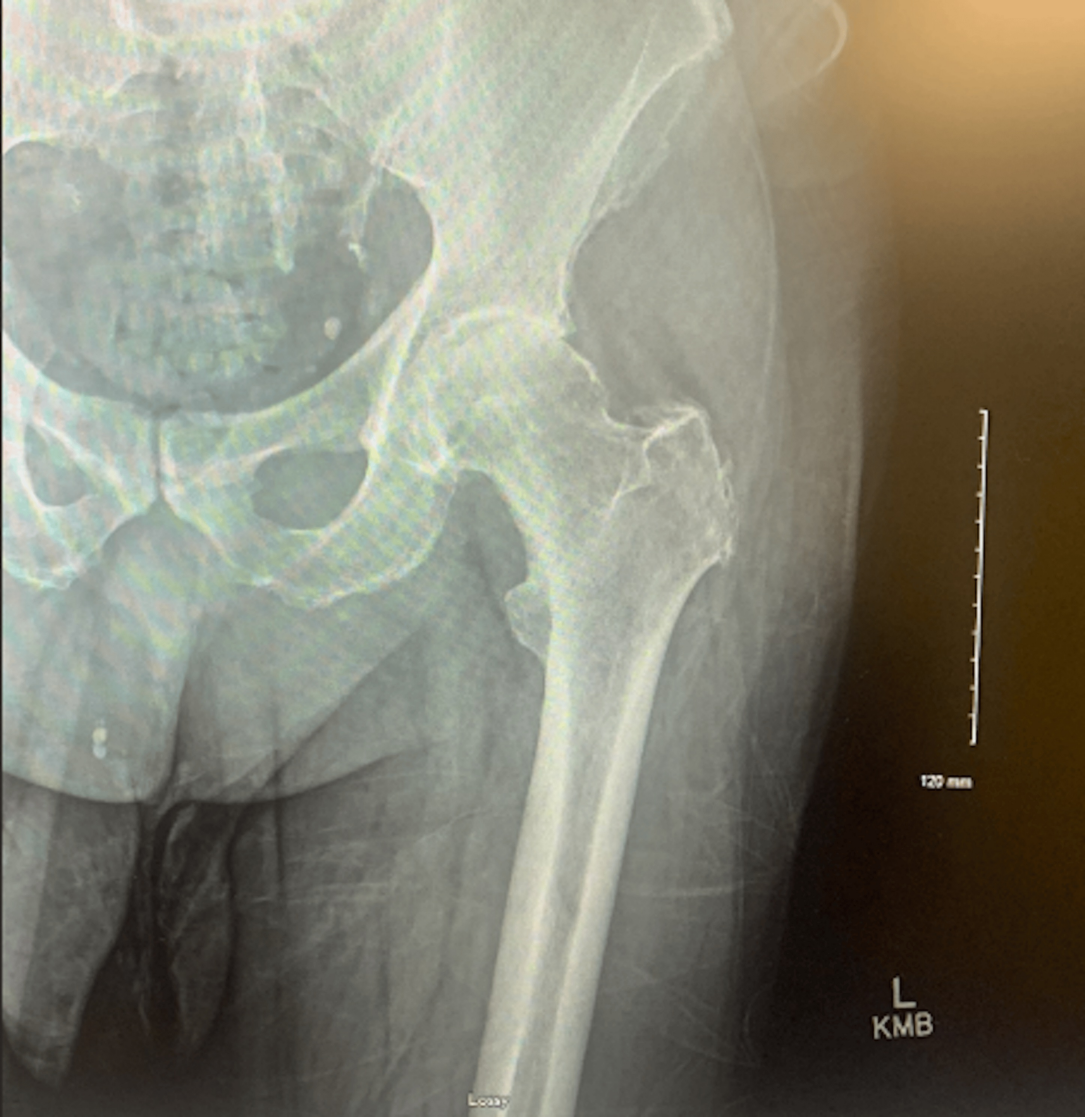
Preoperative anteroposterior radiograph of the left hip
